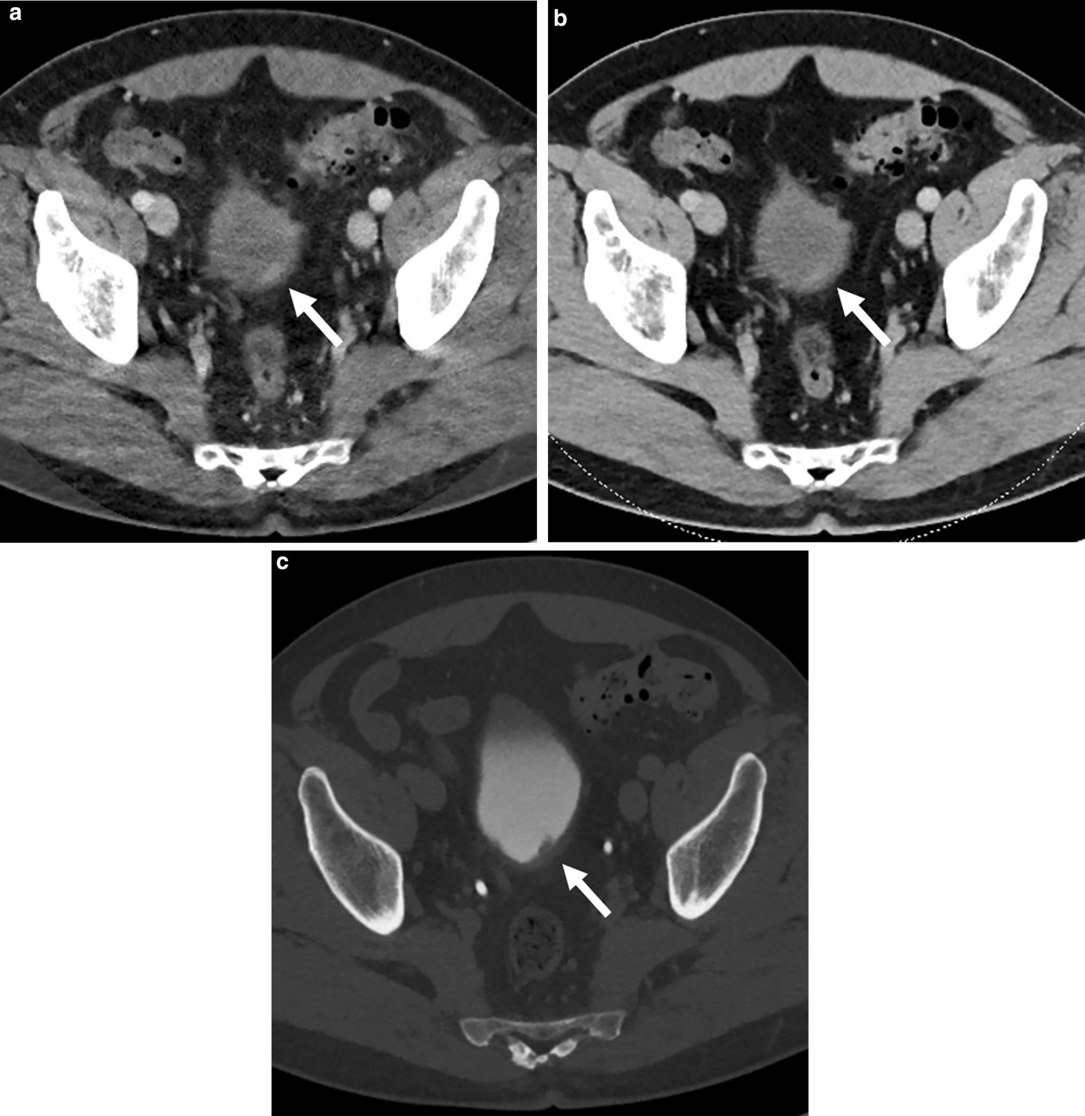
Stage pT1 invasive urothelial carcinoma in a 61-year-old man. Transverse CT images (a 40 keV, b 120 kVp, c excretory phase 120 kVp) show a mass (arrows) on the posterior bladder wall. The mass is more clearly depicted on 40-keV than on 120-kVp images (40 keV, grade 2; 120 kVp, grade 1; excretory phase, grade 3)
